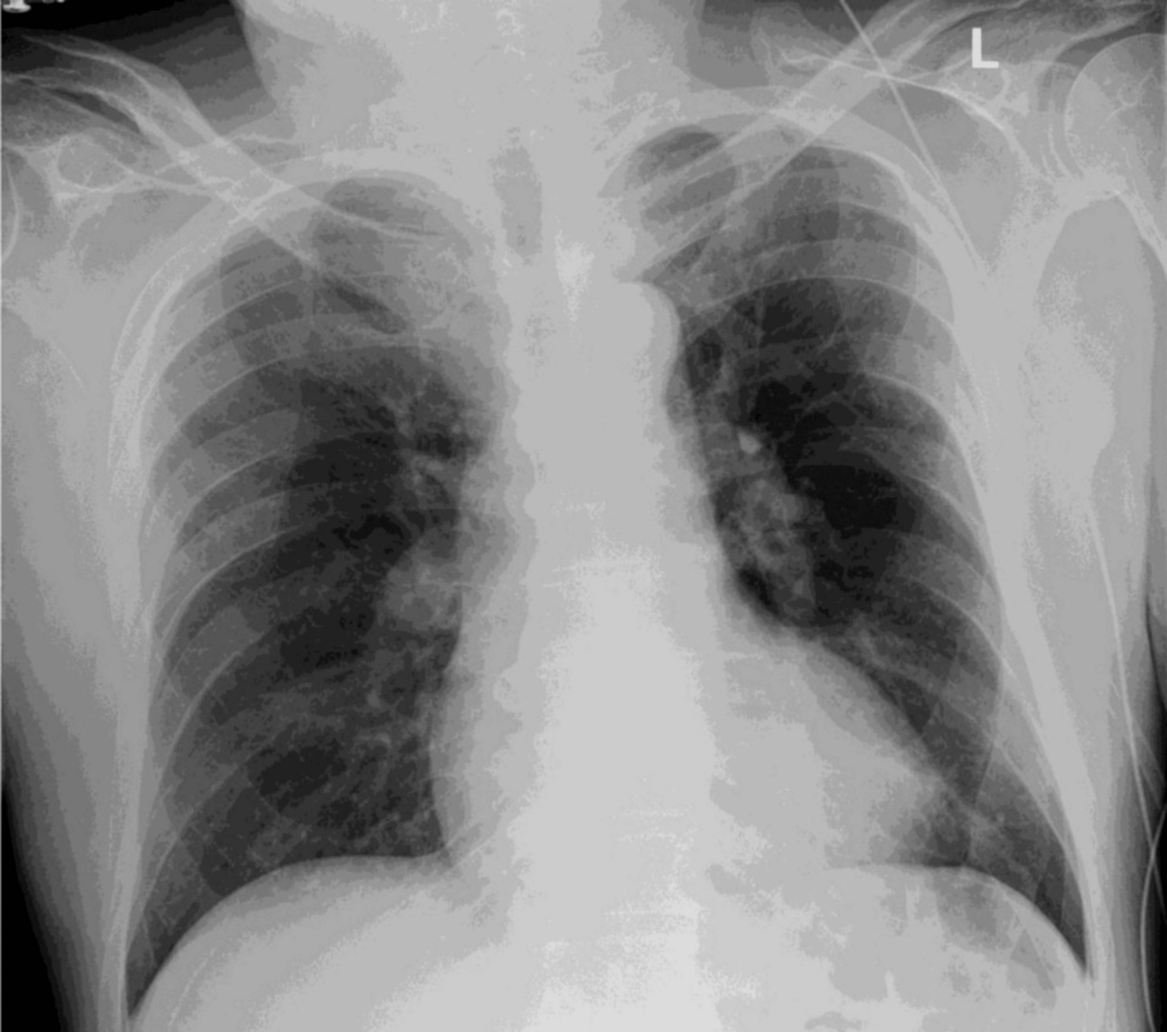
Initial chest radiograph Anteroposterior chest X-ray showing no signs of pulmonary congestion or pleural effusion. Lung fields appear clear, and bony thoracic structures are unremarkable. The cardiomediastinal and hilar shadows appear normal
